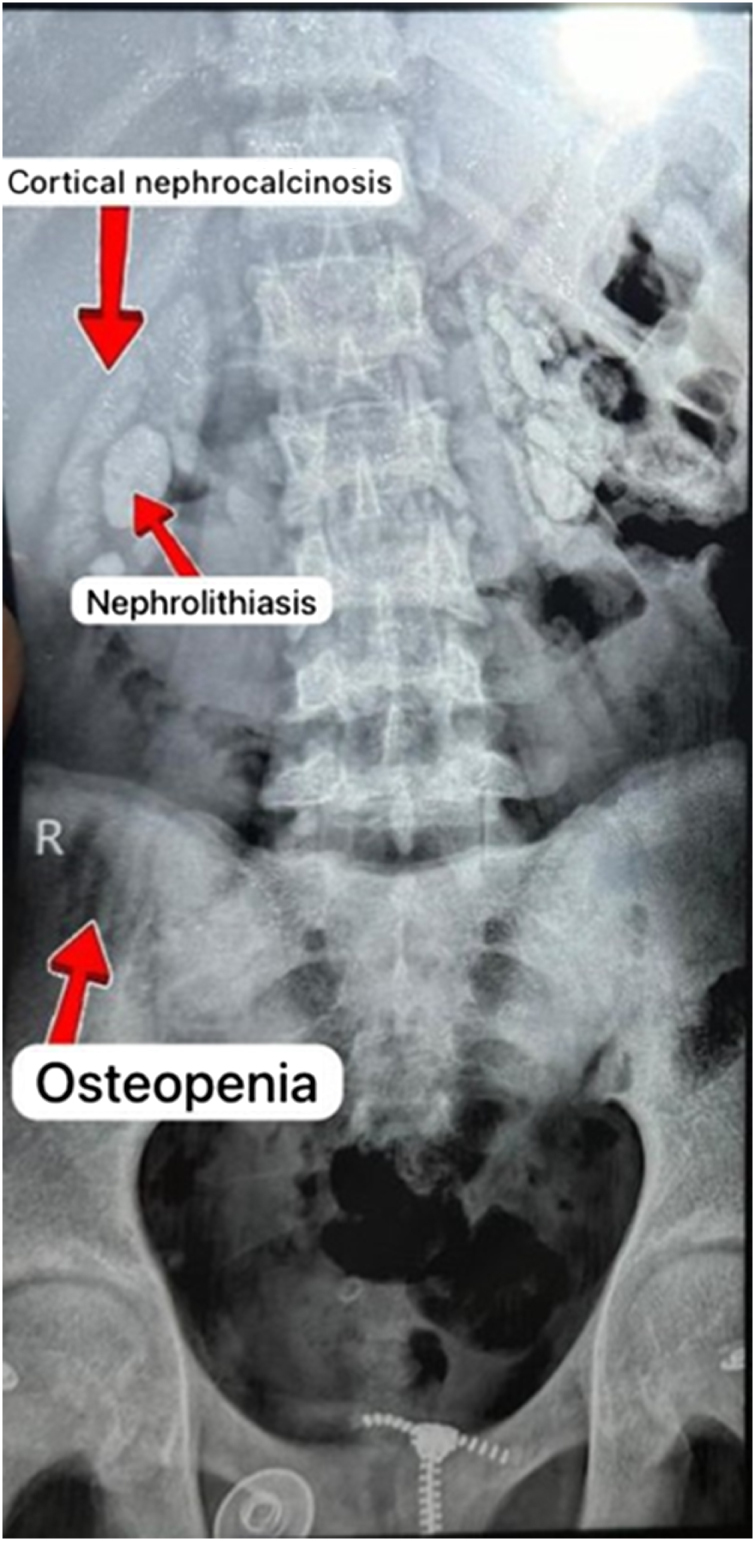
X-ray showing evidence of osteopenia.
